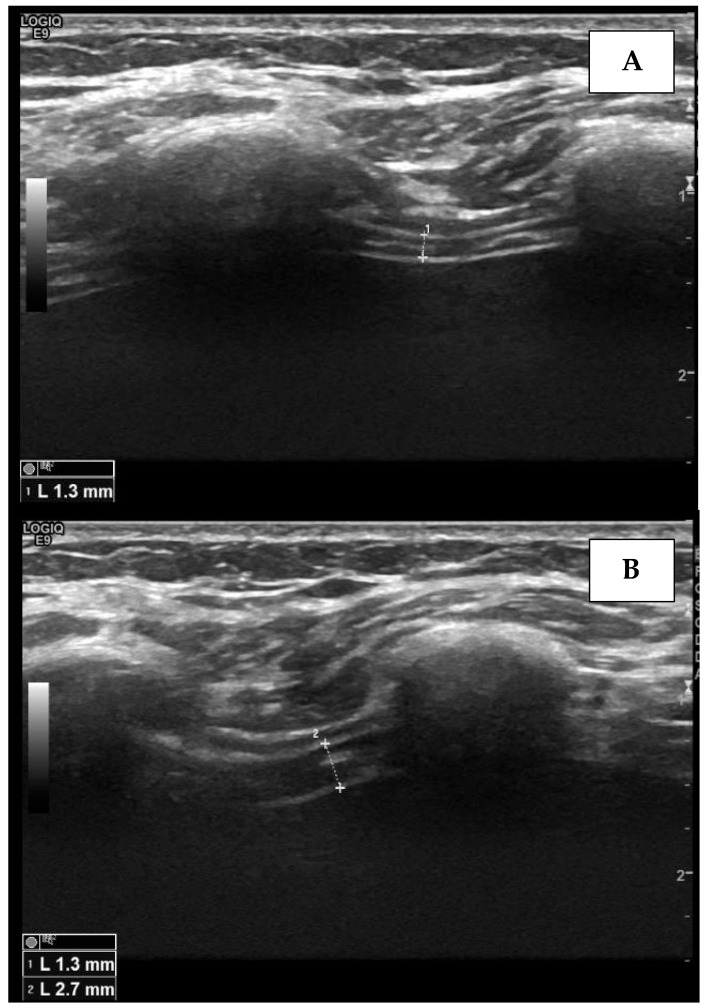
Measurement of the right diaphragm thickness (dotted line) in the end-expiratory phase (A) and end-inspiratory phase (B), using ultrasound. The diaphragm is visualized as a hypo-echogenic central layer surrounded by two hyper-echogenic lines, namely, the pleural line and the peritoneum.
